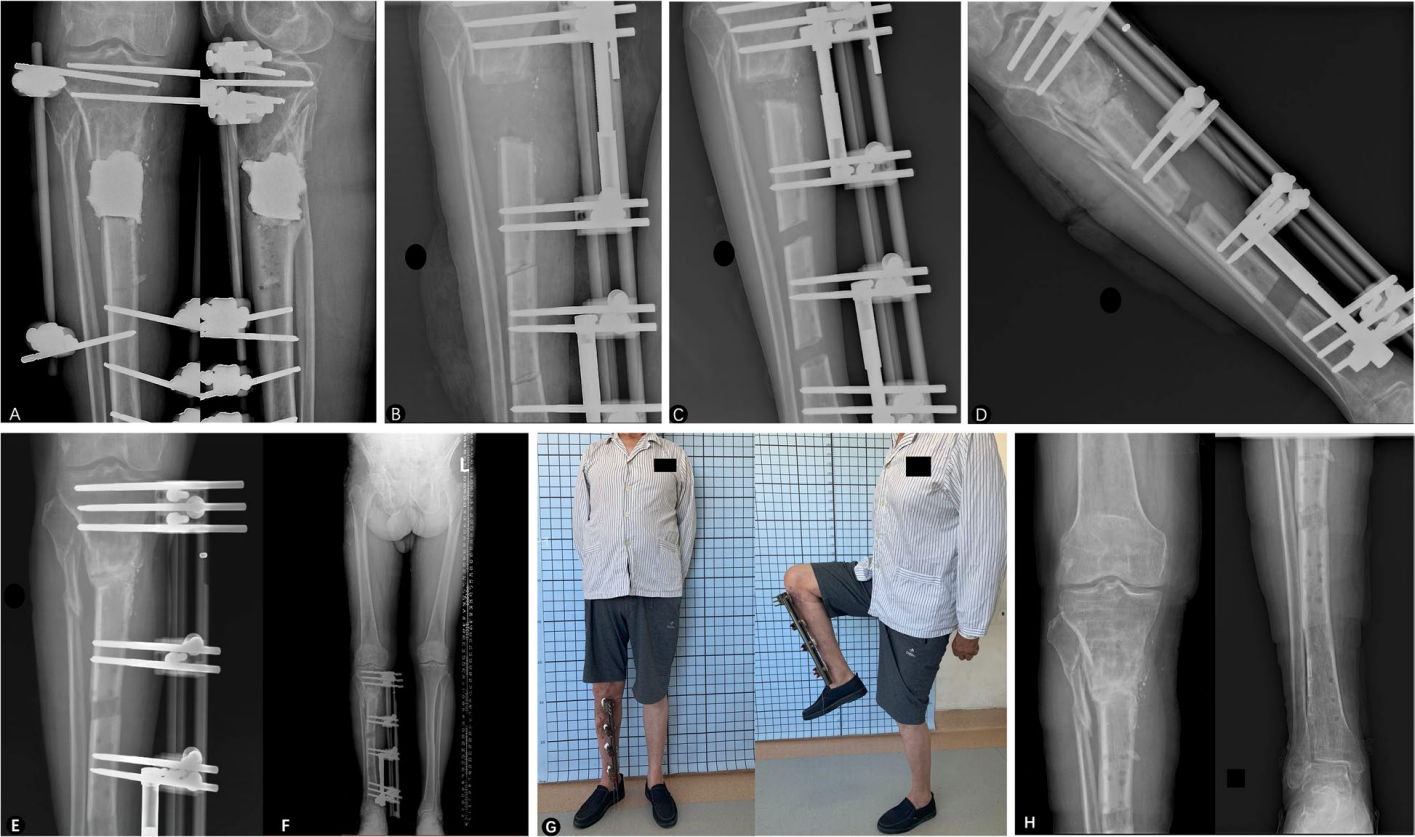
A A 53-year-old male patient with posttraumatic osteomyelitis of the right tibia. After previous debridement operations, there was a defect of 6.1 cm and filled with PMMA spacer. B,C The PMMA spacer was removed 4 weeks later and the trifocal bone transport was started. D Docking was reached at 42 days after bone transport on X-ray. E,F Satisfied regenerates on both distracted area and docking union were achieved 92 days the docking contact. G General appearance before the removal of the external fixator with excellent functional result. H Radiograph appearance with an excellent bone result at 26 months after the removal of external fixator
